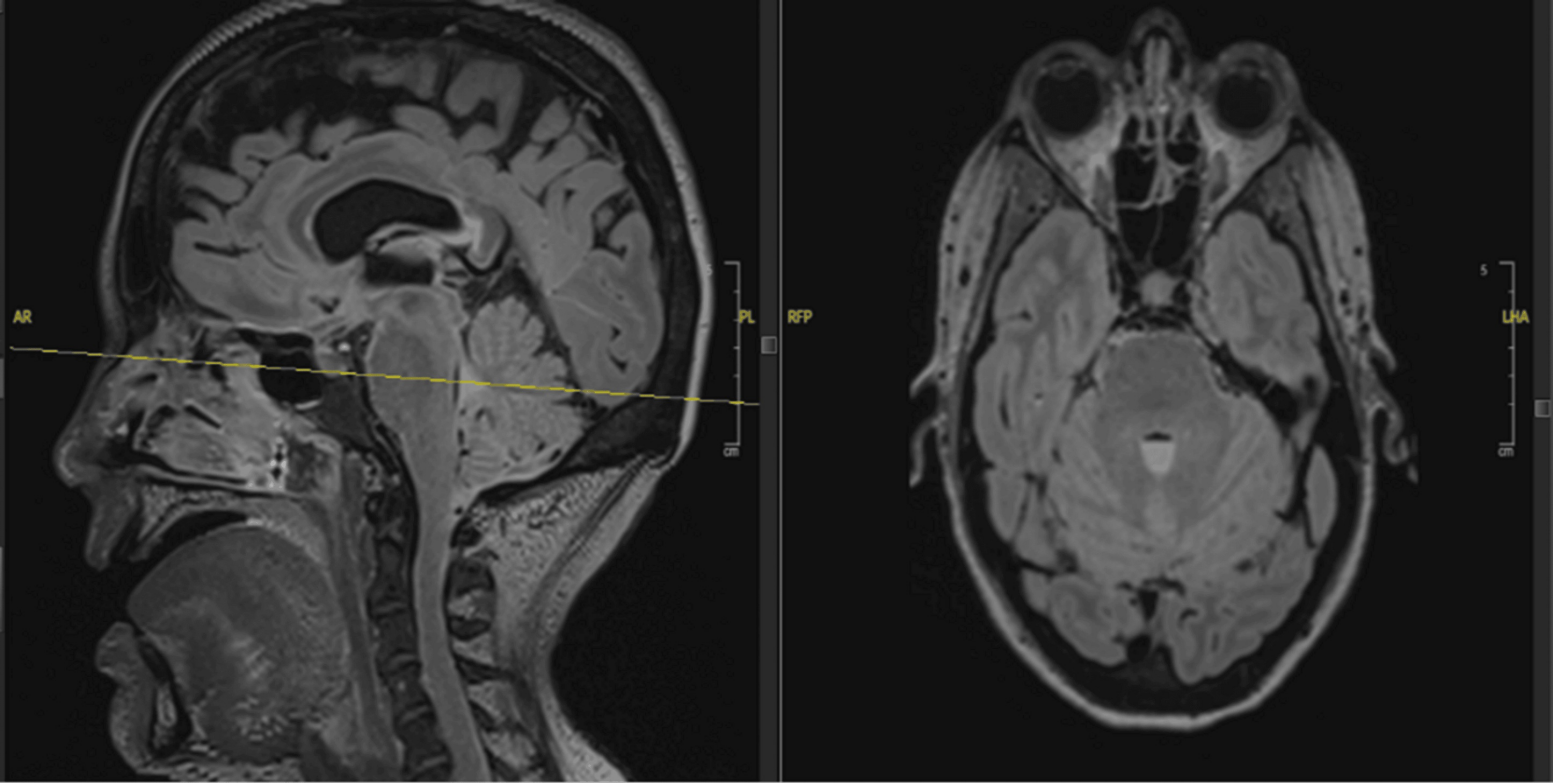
The sagittal plane (left) and transverse plane (right) of the MRI revealed ventriculitis with fluid level in the fourth ventricle.
